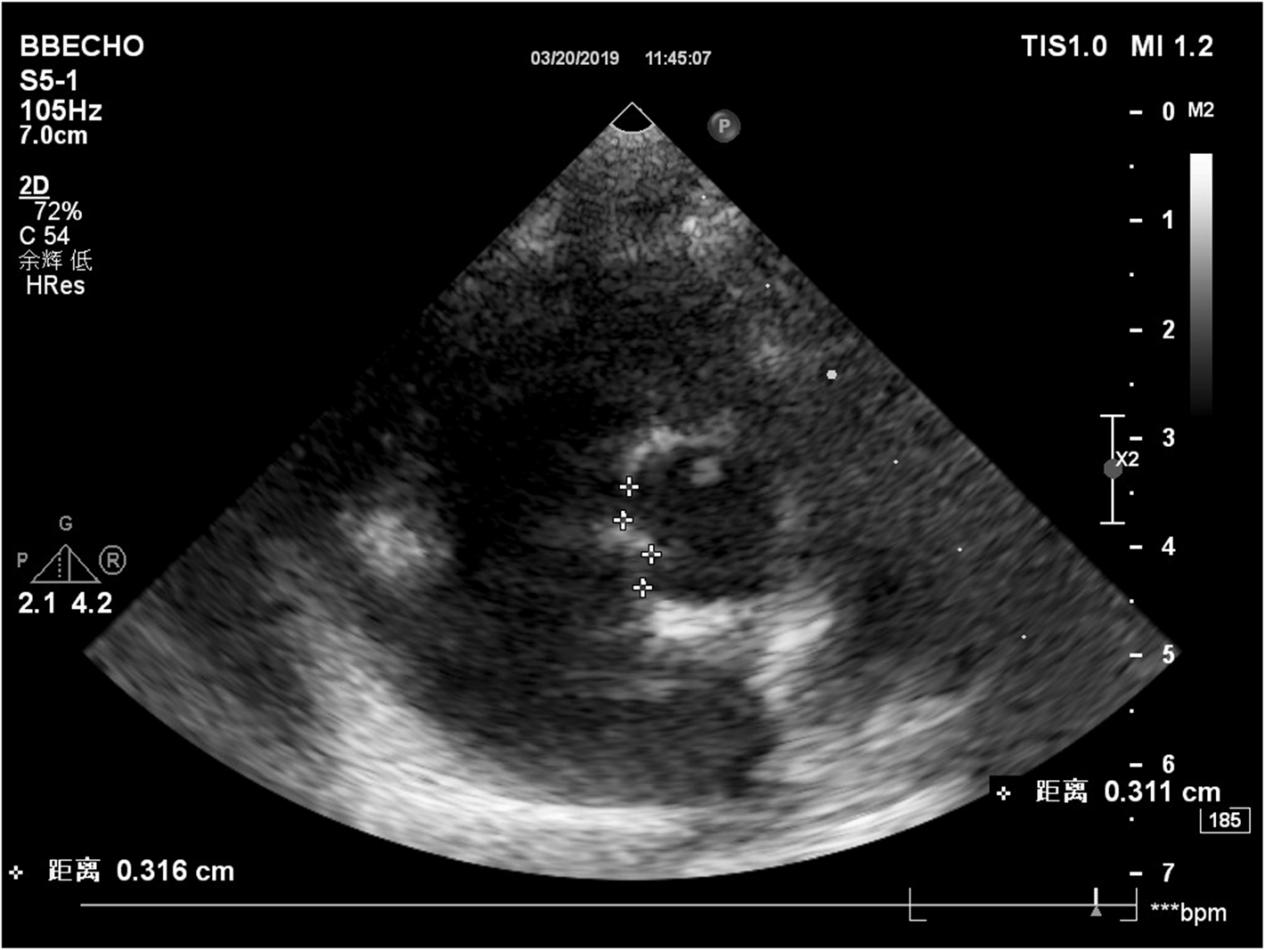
Right CA aneurysm (3.1 mm) was detected on echocardiography on day 5 of hospitalization
